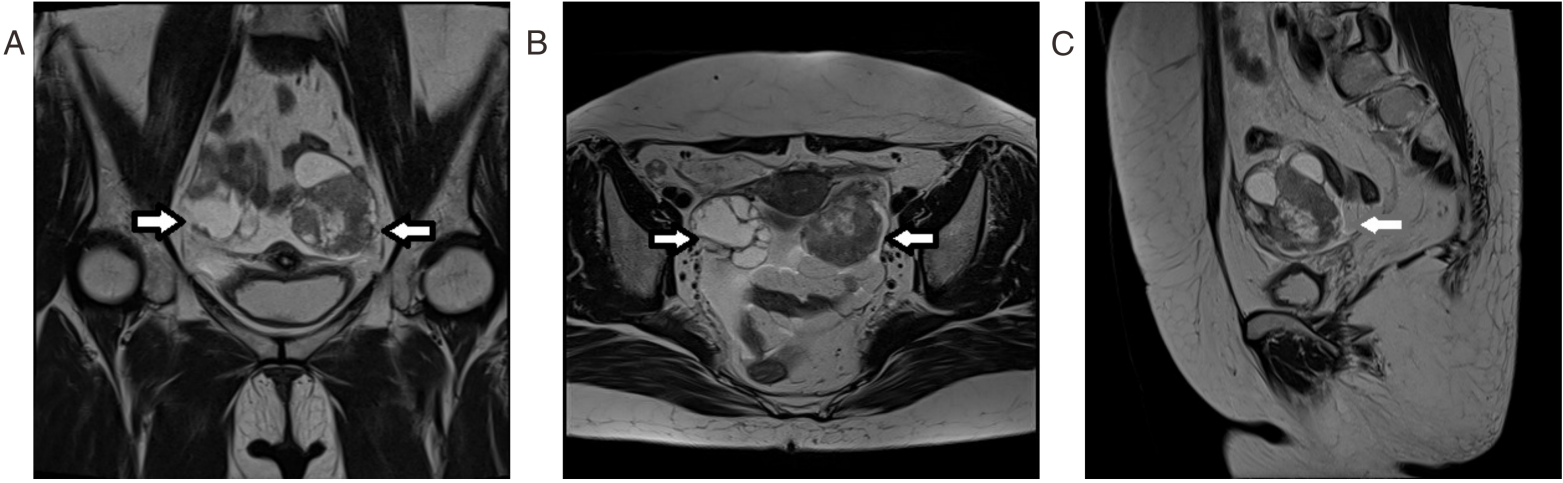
MRI findings of the pelvis:(A) Coronal plane, (B) Axial plane (C) Sagittal plane. Bilateral adnexal regions demonstrate cystic-solid lesions.
